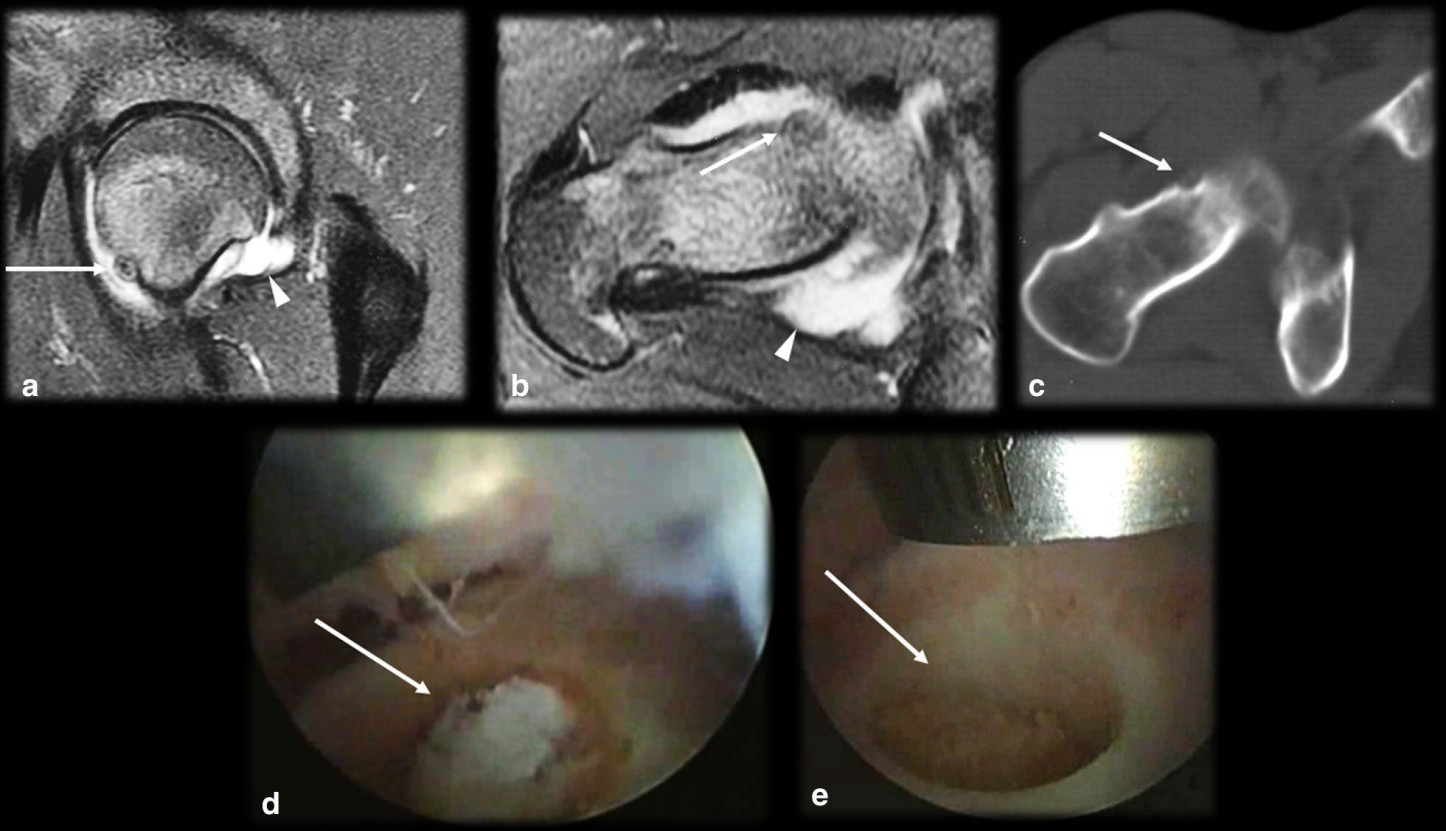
Osteoid osteoma mimicking synovitis of the hip. A 24-year-old man with right hip pain, swelling and tenderness for 2 weeks. Inflammatory marker levels were also elevated. Orthopedists suspected inflammatory arthropathy, and ultrasound (not shown) depicted joint effusion and synovitis. MRI T2 FS sagittal (a) and axial (b) shows joint effusion (arrowhead) with synovial thickening and a doubtful nidus (arrow), which was better characterized on the CT scan (c). Arthroscopic aspect before (d) and after (e) nidus resection
